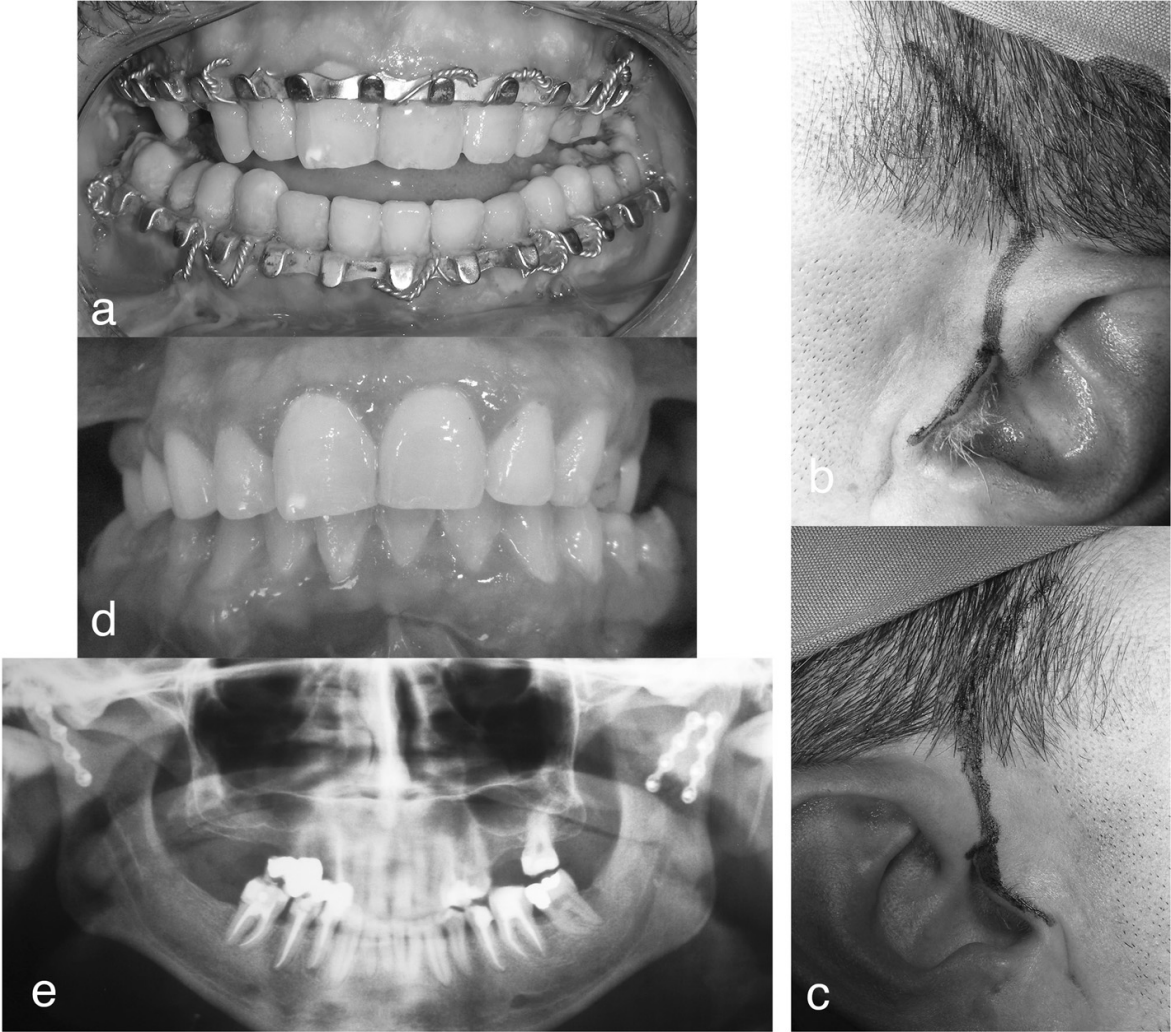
Mandibular sub-condylar bilateral fracture: a) anterior open-bite with a posterior precontact; b) left preauricolar approach; c) right preauricolar approach; d) post-surgey occlusal plane; e)post-surgery orthopantomography.
